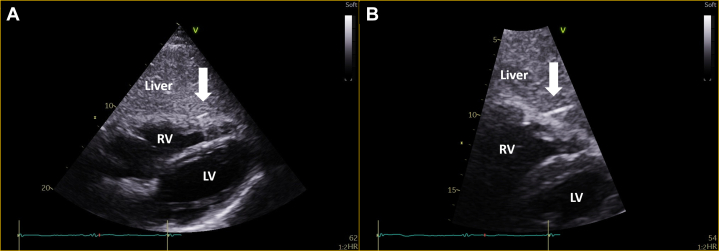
Transthoracic Echocardiography Transthoracic echocardiogram in subcostal windows (A) and (B) demonstrating the foreign body (white arrow) embedded in the liver, in close proximity to the right ventricle free wall. LV = left ventricle; RV = right ventricle.
